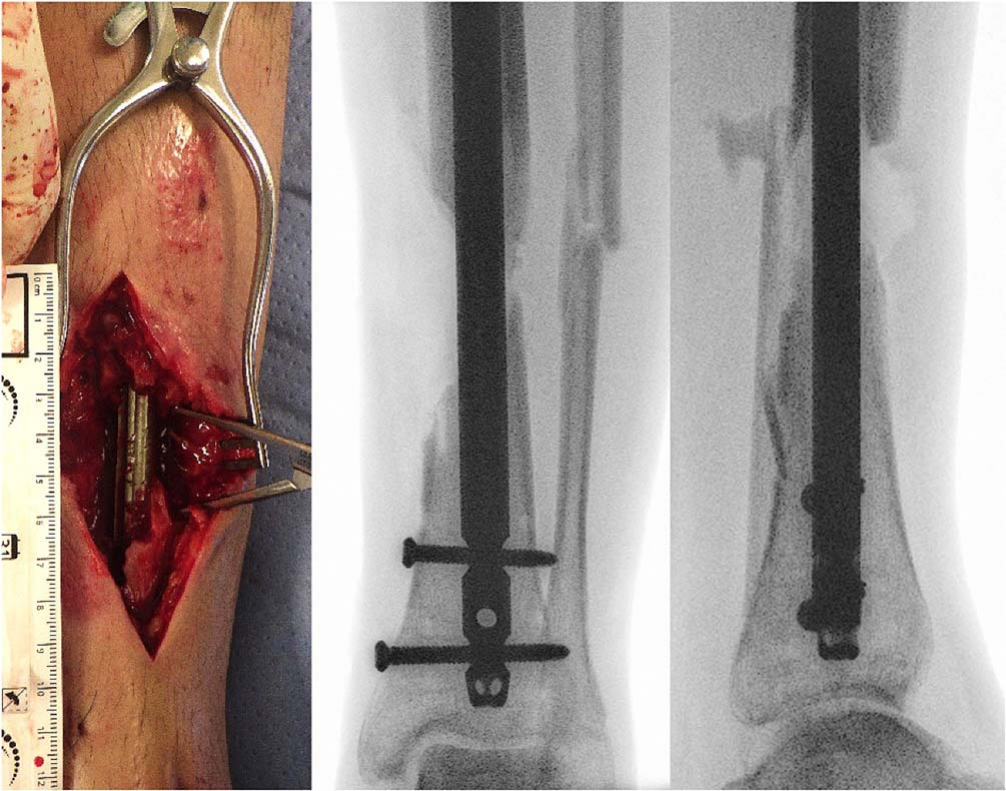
(a) Photography of intraoperative bone defect before graft cage implantation and (b) intraoperative X-rays of left lower extremity show segmental bone defect.
